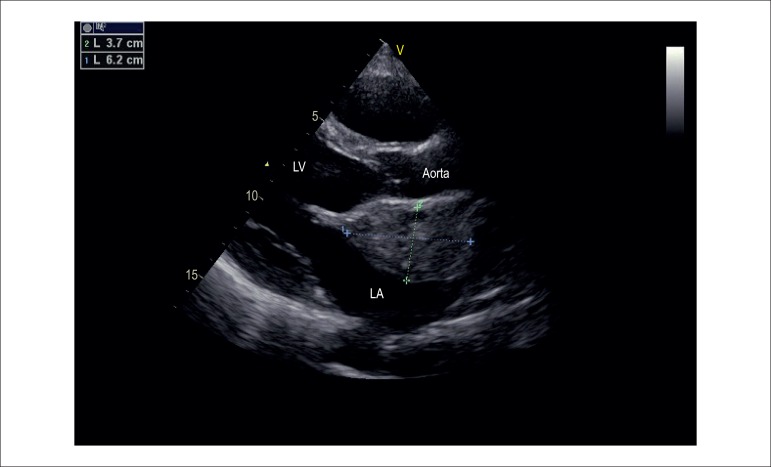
Transthoracic Echocardiography with image suggestive of left atrial
